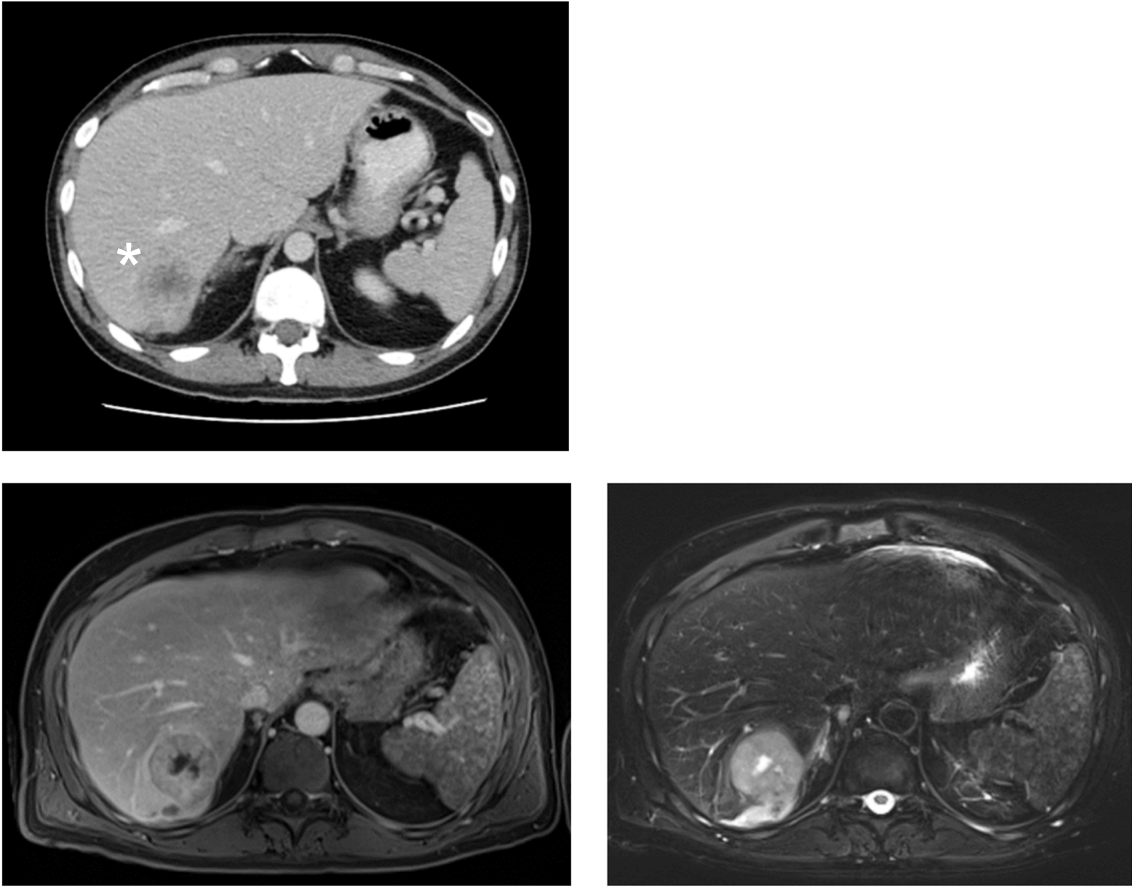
The initial CT abdomen during the portal venous phase in axial reconstruction (upper left) shows a hypodense lesion (*) in liver segment VII. In the MRI examination, the lesion had hypointense signal in the T1 vibe Dixon sequence (lower row on the left) and a hyperintensive signal in the T2 blade fs (bottom row on the right)
